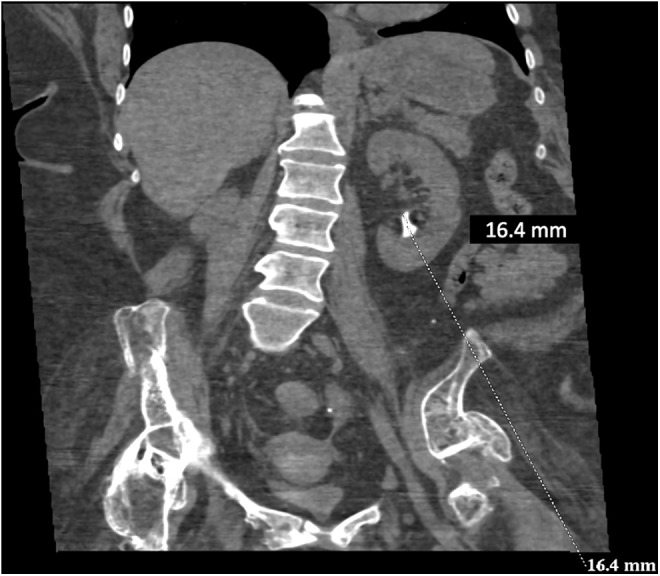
CT scan showing the left renal stone.
